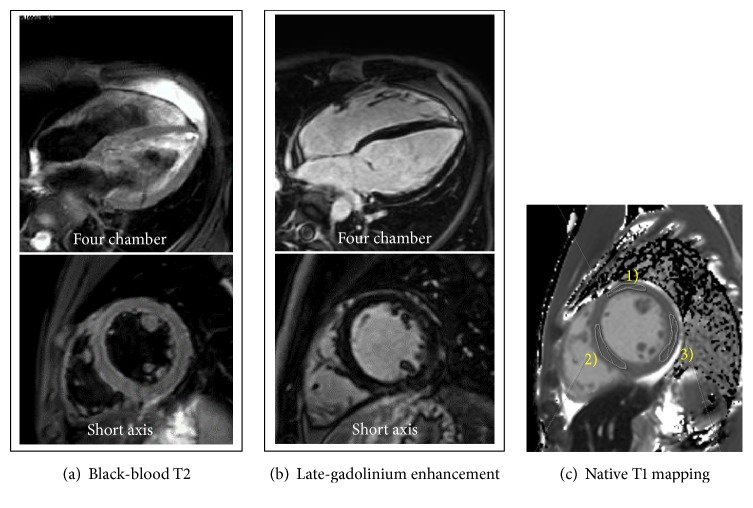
Cardiac 1.5-T magnetic resonance imaging 4 weeks after admission*. (a) T2-weighted black-blood images show no significant high-intensity signal. (b) Late-gadolinium enhancement shows no significant focal high-intensity signal. (c) Native T1 mapping analysis shows mildly elevated values: (1) anterior wall, 1193.01±36.34 msec, (2) septal wall, 1193.05±32.03 msec, and (3) posterolateral wall, 1198.87±42.86 msec.
